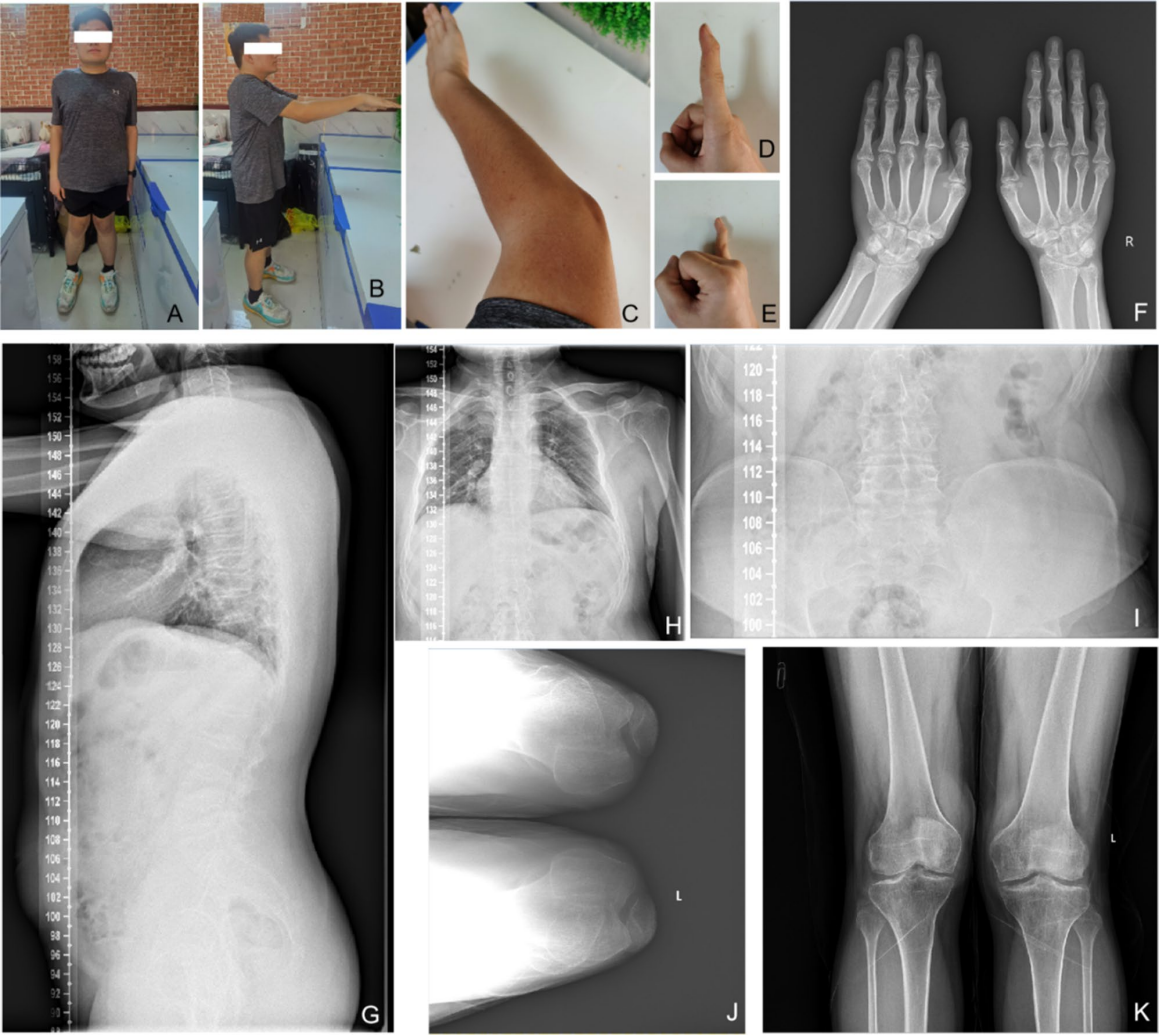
Clinical and radiological phenotypes of the patient with SMDK. A-B. The gross appearance of patient. C-E. The gross appearance of right upper limb (C), index finger (D) and little finger (E), showing the flexion deformity of joints. F. The AP radiograph of hands, showing the degeneration of small joints. G-I. The AP and LAT radiograph of spine, showing slight scoliosis, narrowed vertebral bodies, pectus carinatum, and inwardly curved scapulae. J. The axial radiograph of patellar. K. The AP radiograph of knees, showing degeneration of joints and genu varum of left knee
